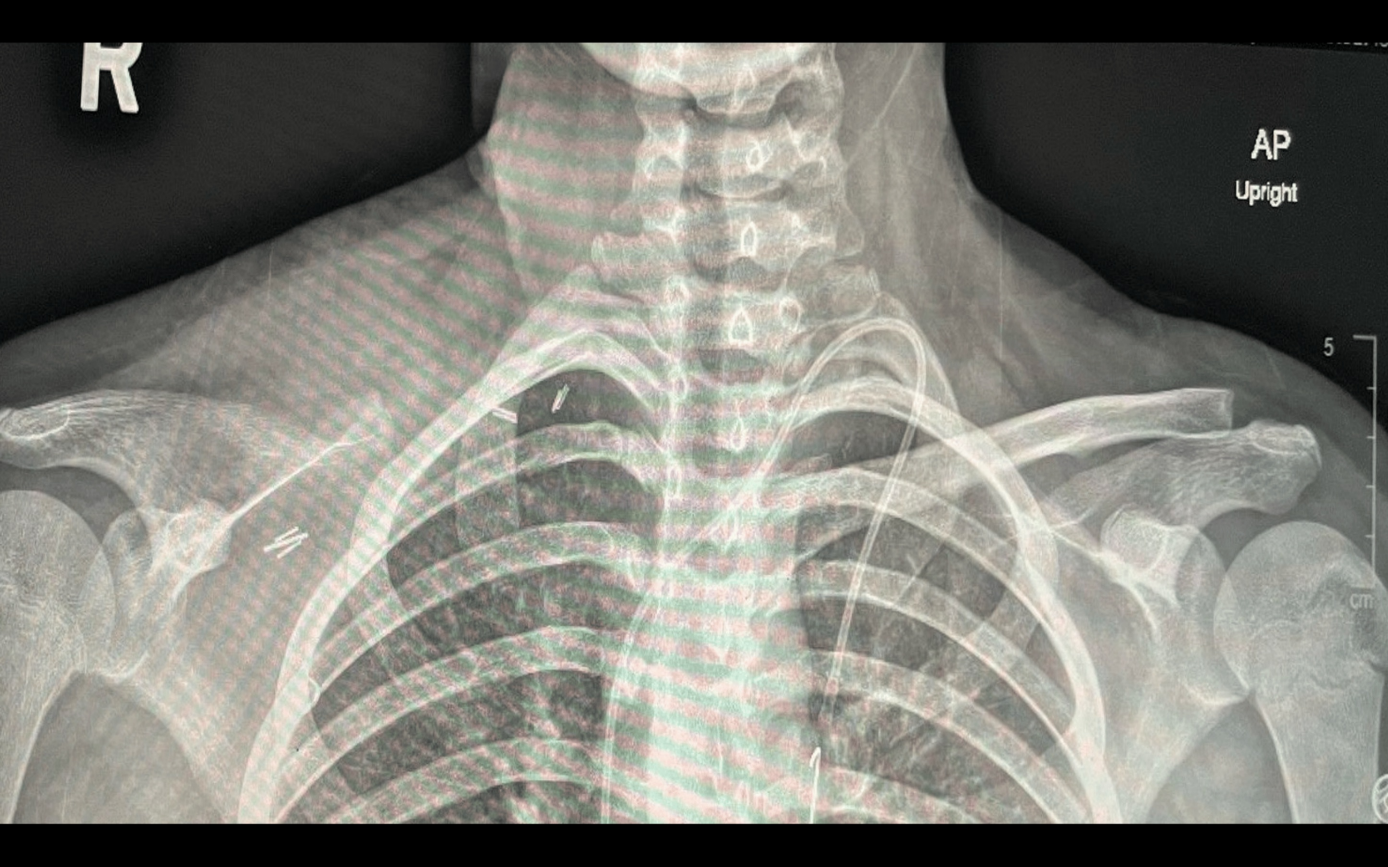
Postoperative X-ray The patient consented to the publication, reproduction, broadcast, and other use of imaging and photos.
